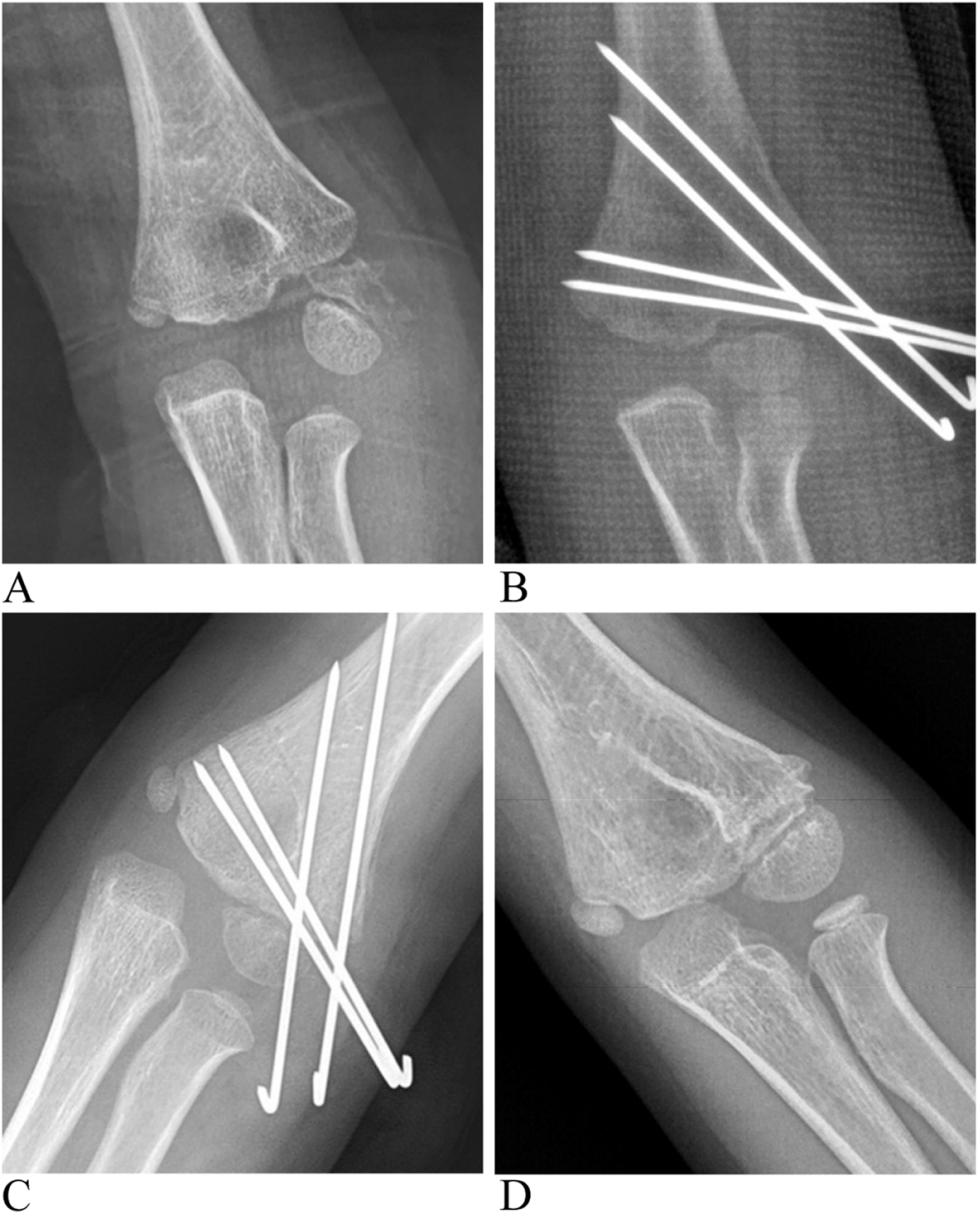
A 5 years old girl with a Song stage 5 and Milch type II LCHF treated with CRPP. A Pre-operative anterior-posterior X-ray. B Post-operative X-ray showed satisfactory reduction. C X-ray 4 weeks post surgery showed well union of fracture. D X-ray 16 months post surgery showed a decreased Baumann angle
